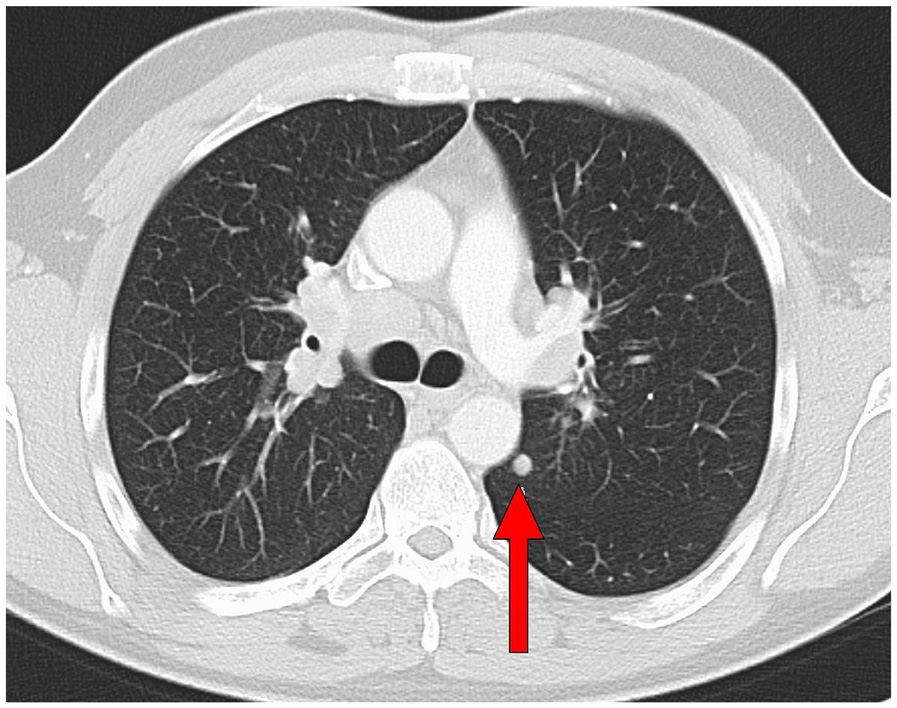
axial slide of a CT-scan of the chest, lung window. Findings before the start of the systemic treatment. Hilar lymphnodes are swollen, the arrow indicates a nodal infiltrate of the left lung.
